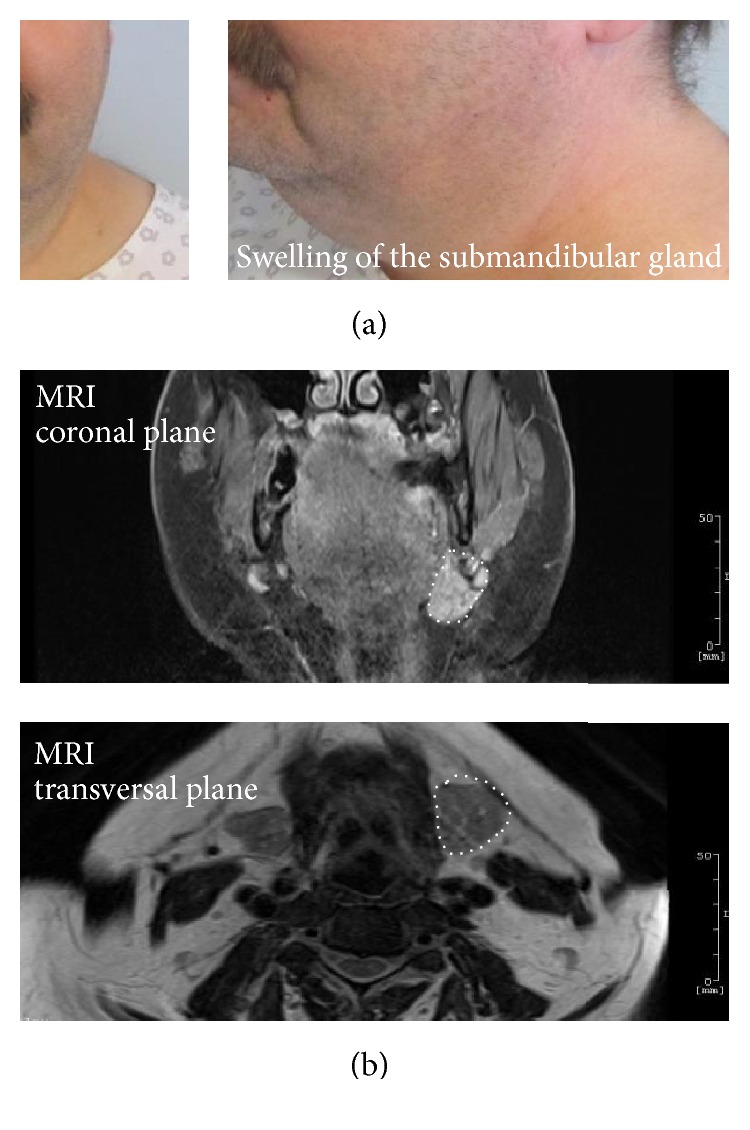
Acute mumps infection involving swelling of the left submandibular gland. (a) Photographs from the left submandibular region of the patient show a superior palpable tumor. (b) MRI reveals an enlarged left submandibular gland with contrast agent accumulation. The left submandibular gland is highlighted by a dotted white line.
